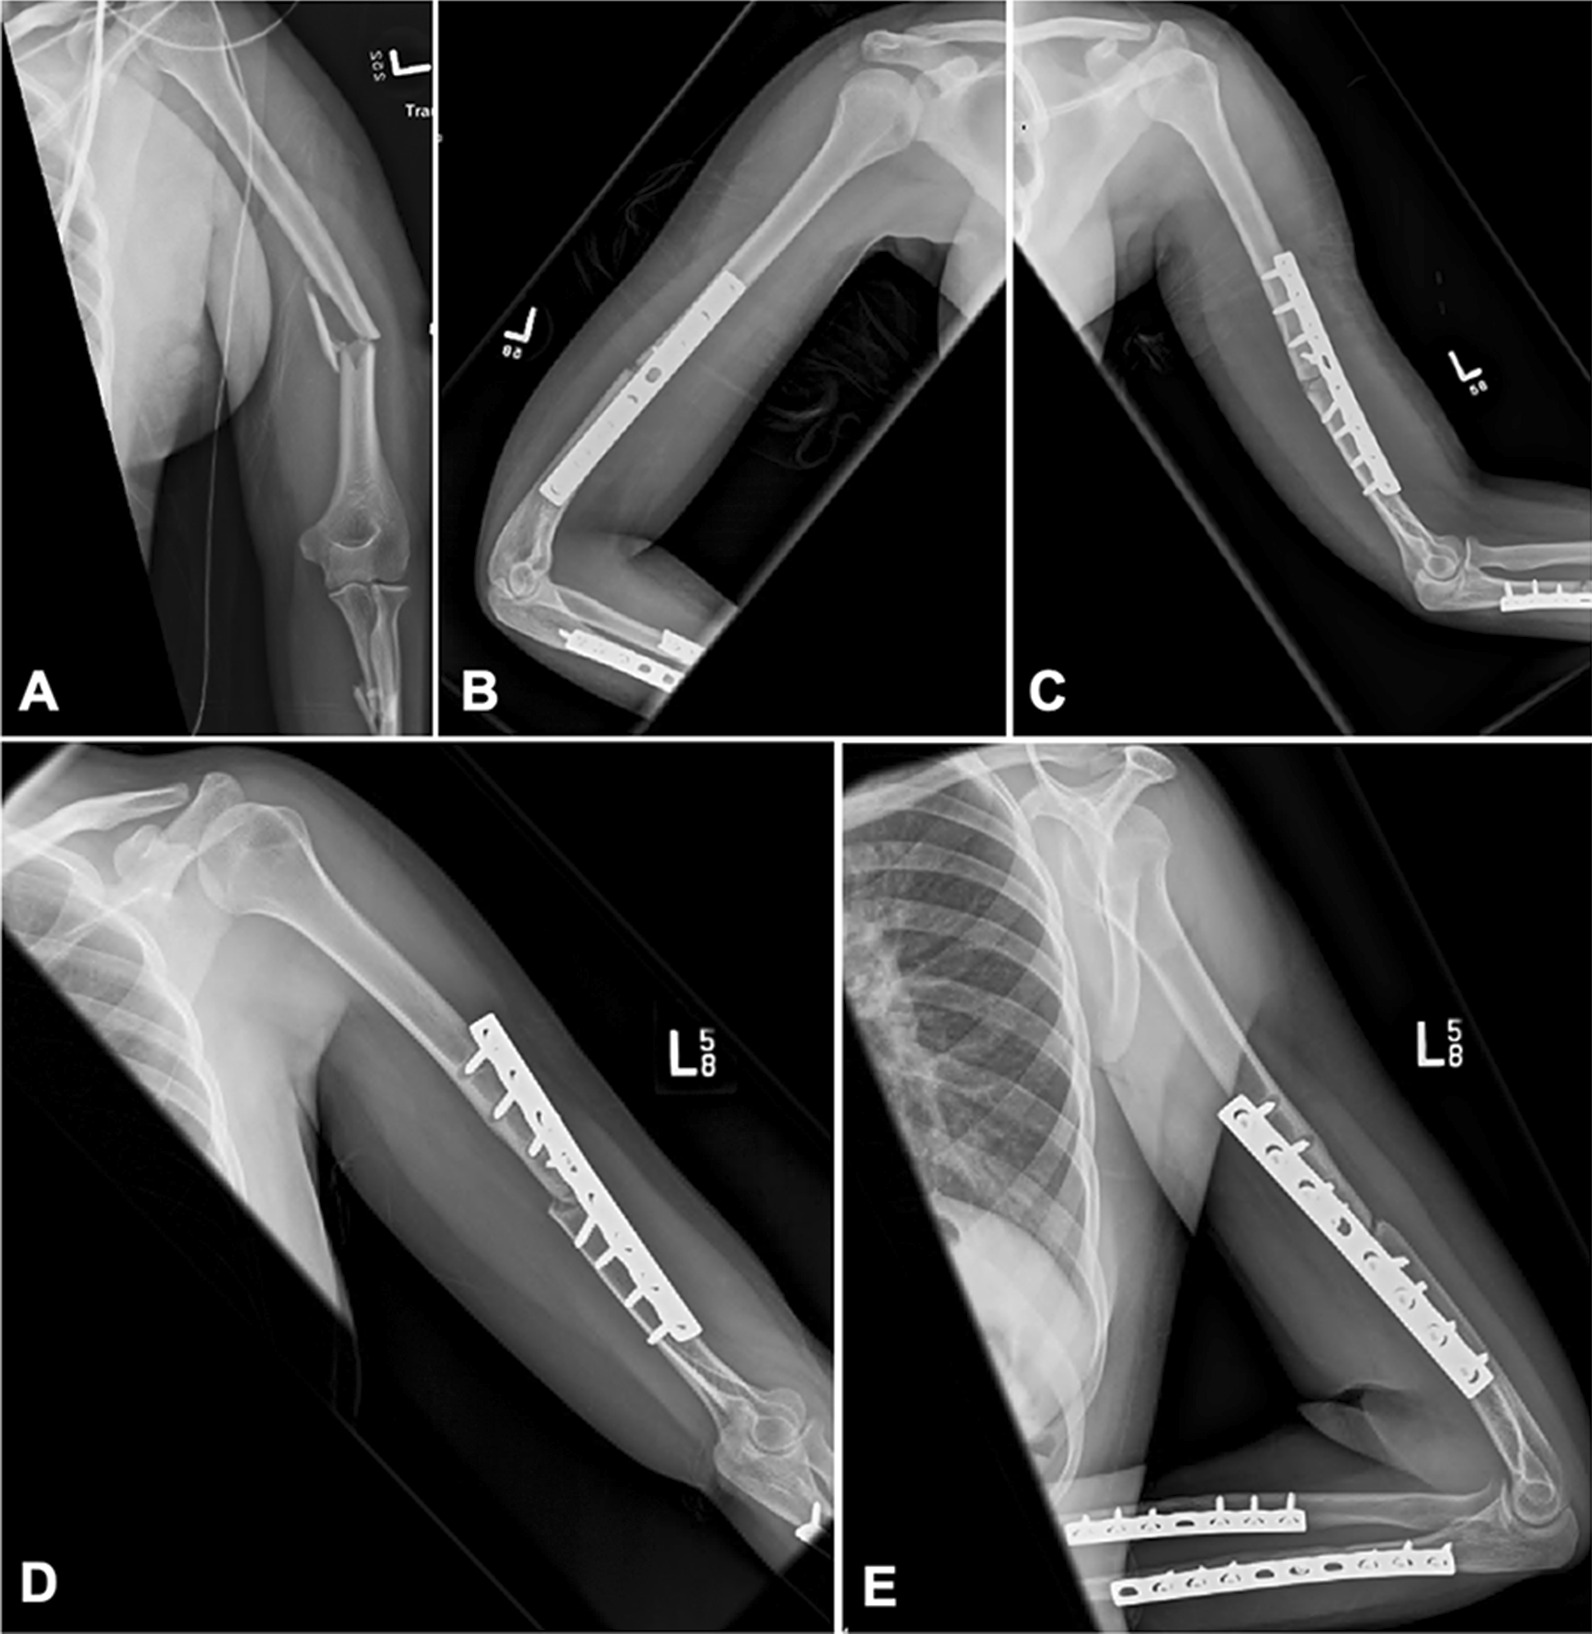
25-year-old female with a middle 1/3 diaphyseal, AO/OTA 12B2 humerus fracture (A). Patient received ORIF with Synthes narrow 4.5 mm LCDC plate, with immediate post-operative radiographs shown (B, C). Radiographs demonstrate unicortical fixation of one of the proximal screws, and 5 cortices of proximal fixation (C). Two years late the patient went on to mechanical failure with proximal screw pullout (D, E), requiring revision
